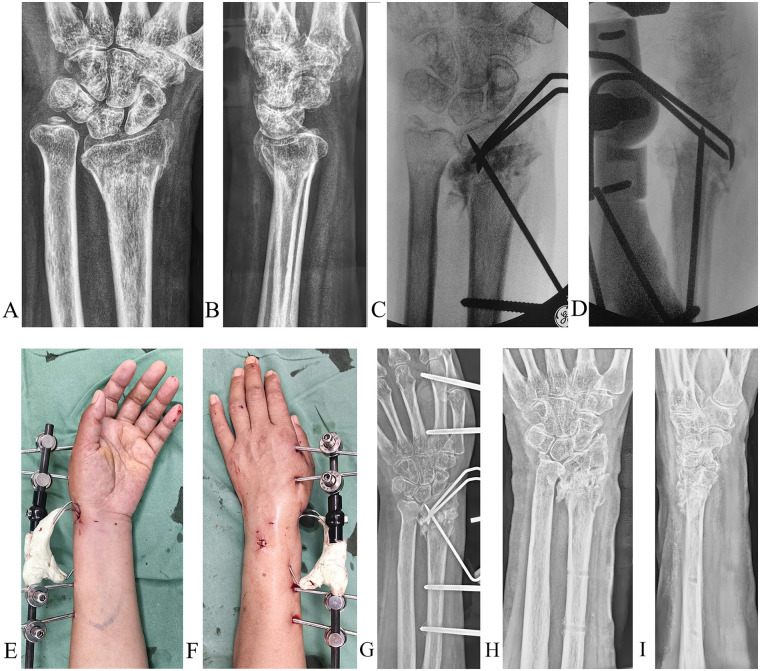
A 37-year-old female patient suffered from depressed distal radial malunion (initial type C3) because of a fracture that occurred 6 months before admission. (A) A preoperative PA X-ray. (B) A lateral X-ray. (C) A PA X-ray taken immediately after correction and bong grafting. (D) A photograph showing the anterior aspect of the injured forearm. (E) A photograph showing the posterior aspect of the forearm. (G) An AP X-ray taken 6 weeks after surgery. (H) A lateral X-ray.
